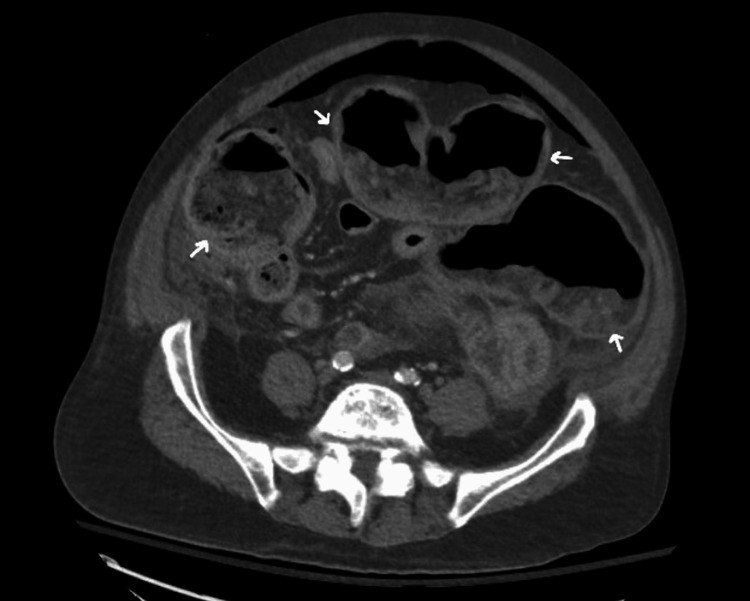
CT abdomen and pelvis with contrast - image 1 White arrows indicate bowel wall thickening consistent with submucosal edema seen in intestinal ischemia. Severe distention of the large bowel with fecal material, fluid and air/gas. There is also free air in the abdominal cavity (pneumoperitoneum) CT: computed tomography
